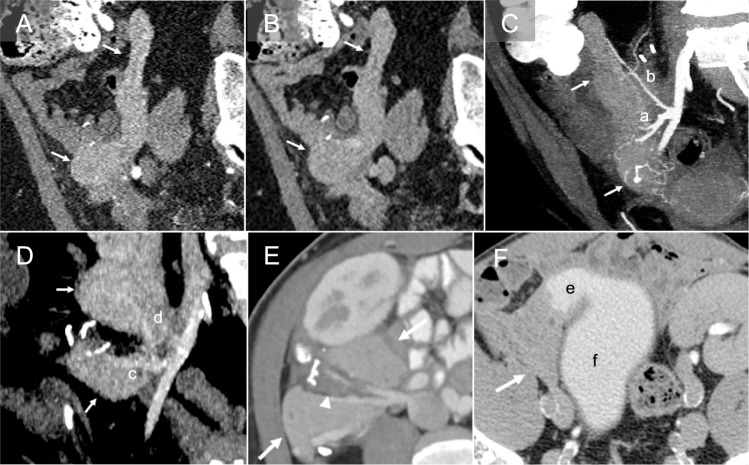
Normal findings on computed tomography (CT). A pancreas graft (arrow) demonstrates homogeneous enhancement during the arterial (A) and delayed (B) phases. CT angiography demonstrates the branching and course of the pancreas graft arteries (C) and veins (D): superior mesenteric artery (a), splenic artery (b), superior mesenteric vein (c), and splenic vein(d). Mild fat stranding in the graft mesentery is likely due to lymphatic edema and is a normal finding in the immediate postoperative setting (arrowhead, E). CT urography (F) shows contrast excretion into the mildly distended duodenal graft (e) and the recipient’s bladder (f) and delineates the anatomy
